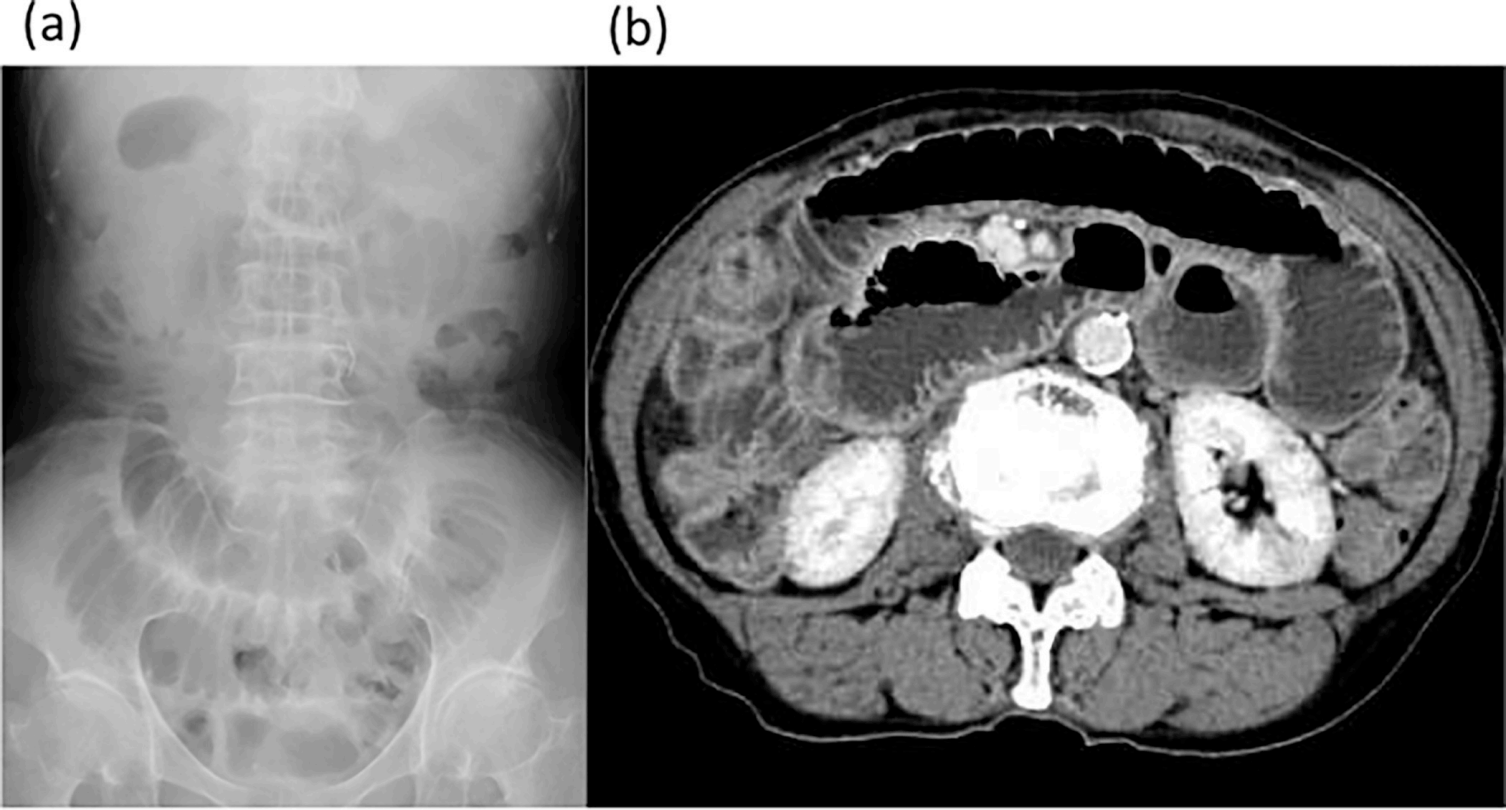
Radiography of acute bowel obstruction. Abdominal plain radiography (a) and abdominal computed tomography (b) of the adhesive small bowel obstruction.
